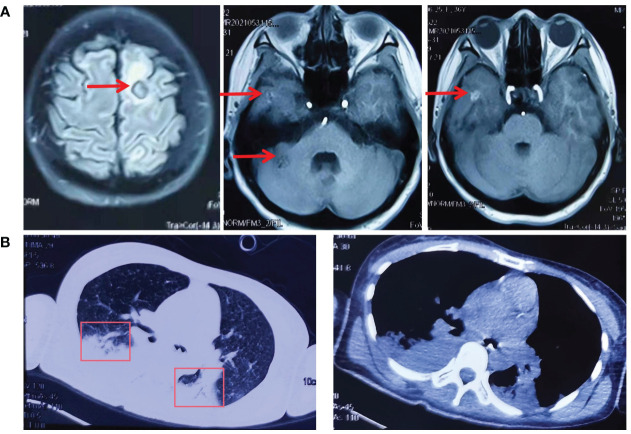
The cranial MR and CT of the chest before and after lazertinib and amivantamab treatment. (A) In May 2021, cranial MR showed multiple abnormal signals in the left frontoparietal-occipital lobe, basal ganglia region, thalamus, and right cerebellar hemisphere; (B) In July 2021, CT of the chest showed pulmonary atelectasis and pleural effusion.
